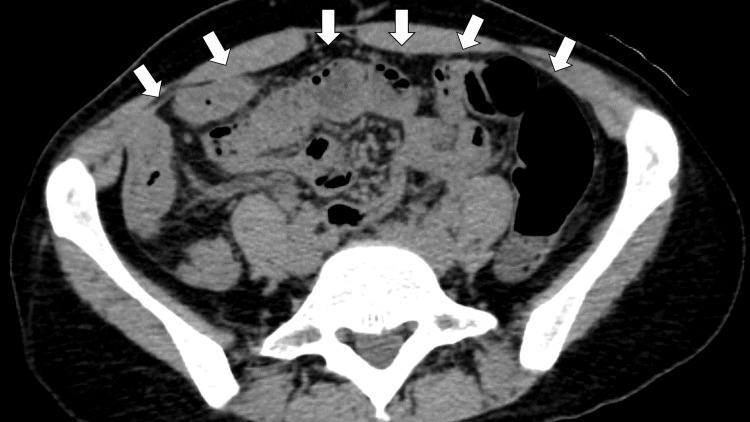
Abdominal computed tomography clarifying whole edematous changes in the small intestine (white arrows)
